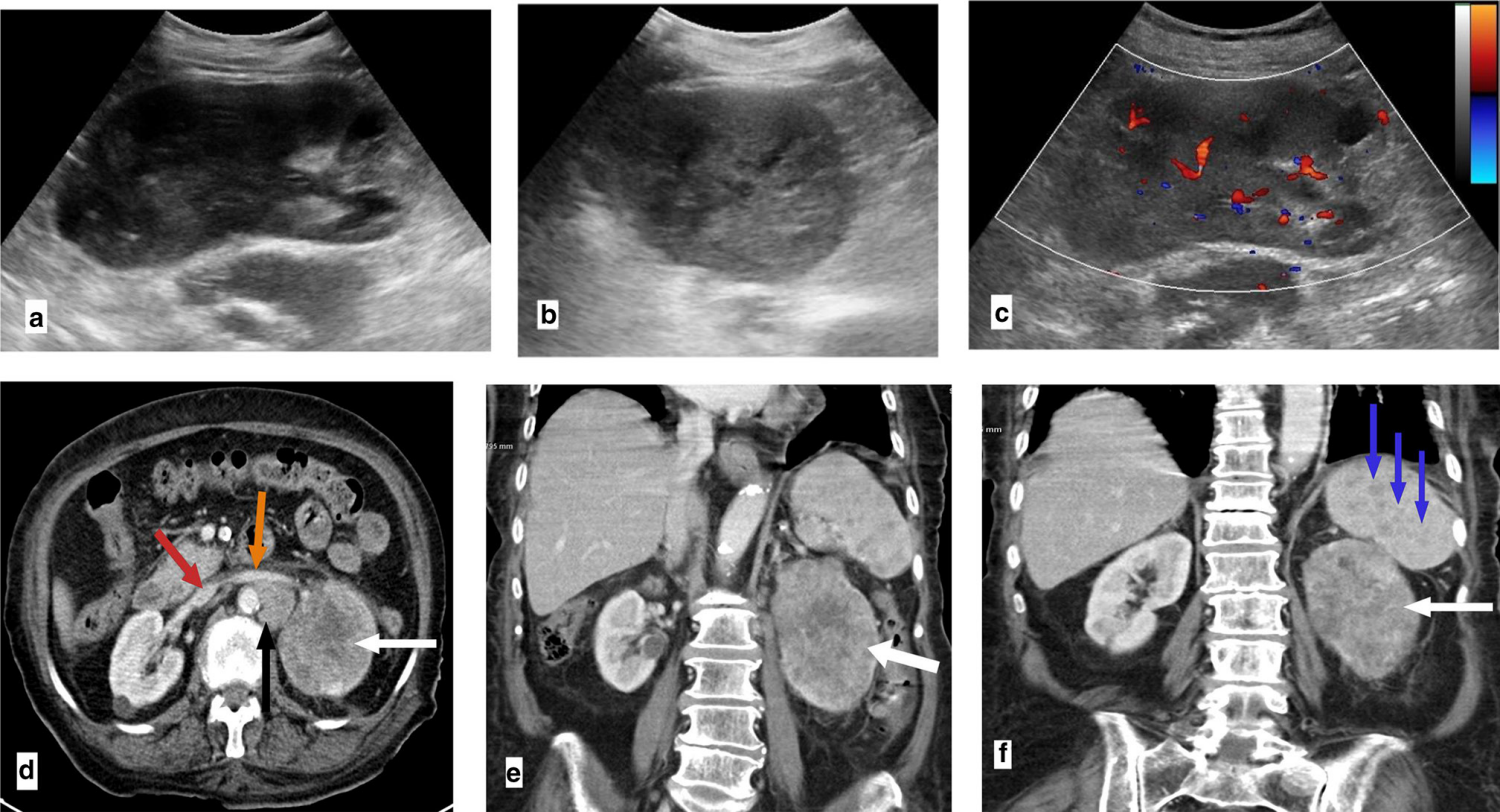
Longitudinal (a) and transverse (b) grey scale US images showing a poorly defined infiltrating heterogeneous hypoechoic lesion at the upper and mid poles of the left kidney. Colour Doppler US examination (c) showed intralesional vascularity. The normal shape of the kidney was preserved. Axial (d) and coronal portovenous (e, f) Phase CT images showing the relatively enlarged left kidney with an infiltrating poorly enhancing mass lesion that almost completely replaces its upper and mid poles (white arrows). Patent left renal vein (orange arrow in D) and IVC (red arrow in D). Enlarged lymph node (black arrow in D) adjacent to the left renal vein. Multiple small focal hypodense lesions in the spleen (blue arrows in F). Abbreviation: US, ultrasound; IVC, inferior vena cava.
